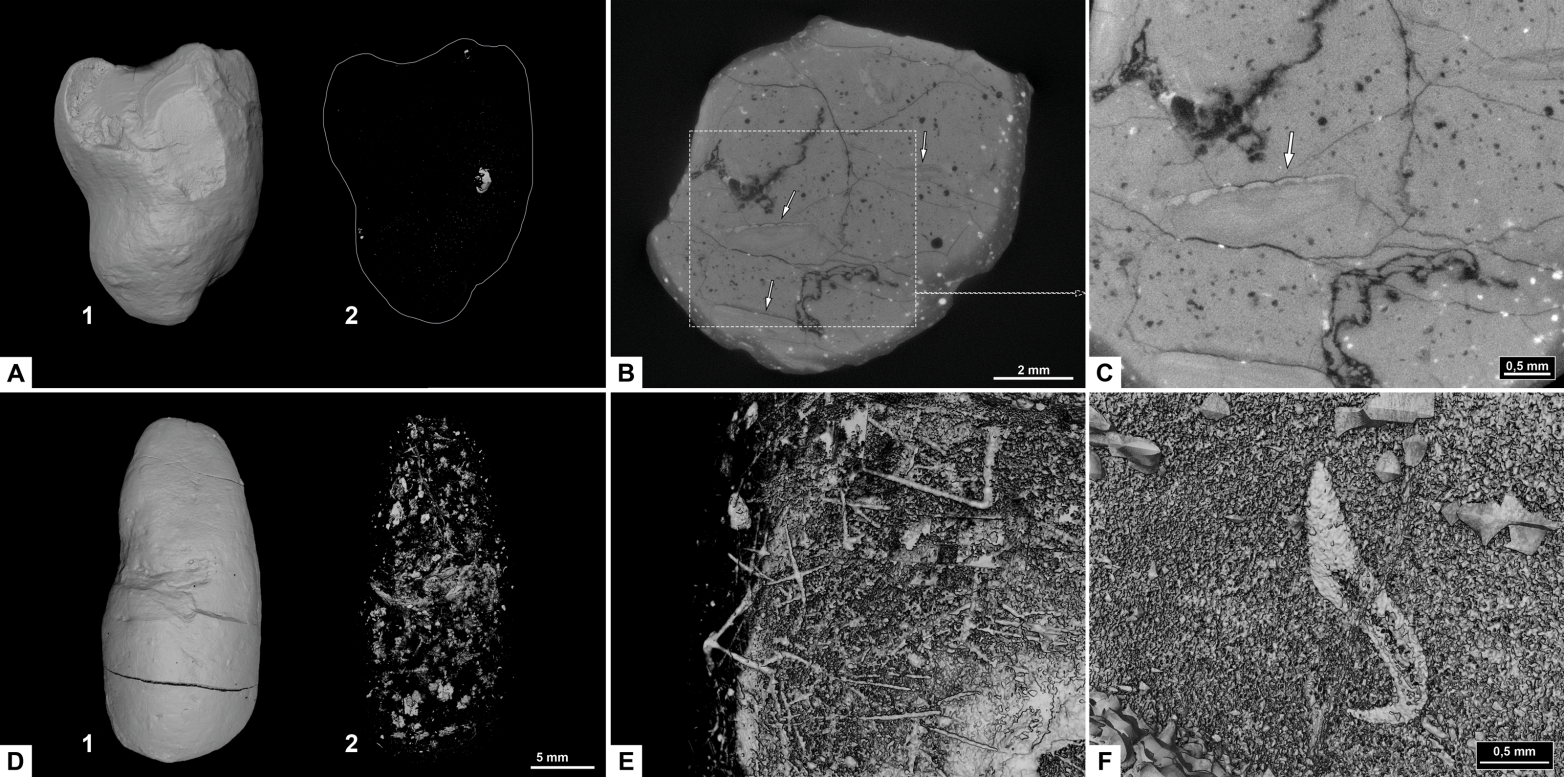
CT-scans of morphotype B. (A1–A2) External and internal view of sub-morphotype B1, PMO 250.533. (B) Orthoslice with white arrows pointing to fish scales, PMO 250.241. (C) Orthoslice with arrow pointing to fish scale with ridges, PMO 250.241. (D) External and internal view of sub-morphotype B2, PMO 250.265. (E) Sponge spicules measuring 0.5–1.0 mm, PMO 250.265. (F) Inverted CT-reconstruction of onychite measuring 2.3 mm in length, PMO 250.265.
